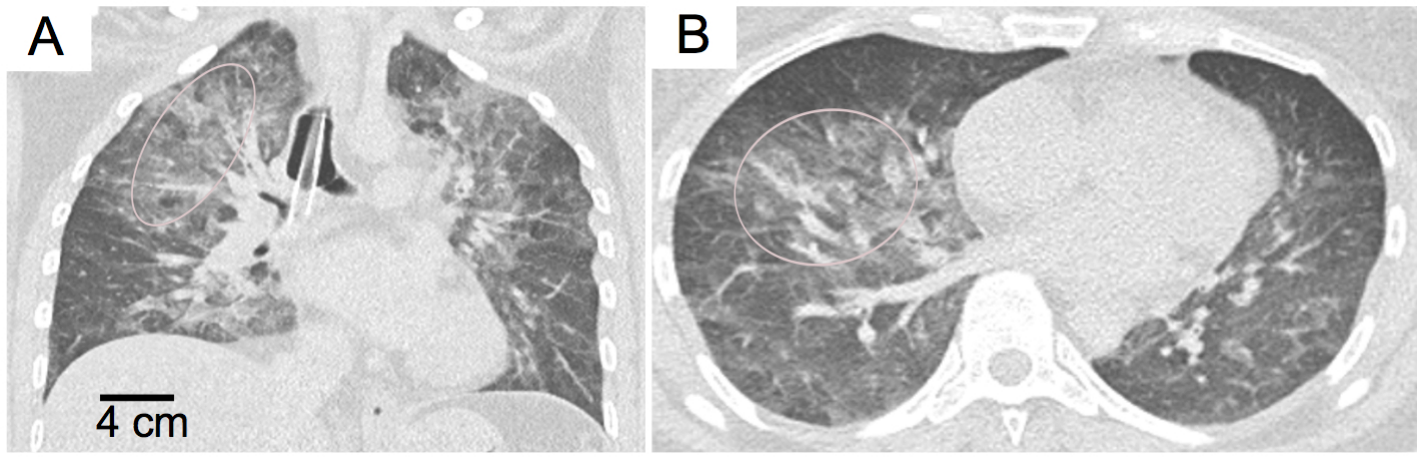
Computed tomography (CT) human chest images of a deceased body showing pulmonary edema due to cardiac failure. Coronal CT image (A) at a level dorsal to the tracheal bifurcation and axial CT image (B) of the lower lobes. The pulmonary edema indicated by the markers presents as diffuse ground-glass opacities with a predominant perihilar distribution. Both images: 3 mm slice thickness, window level -600 HU, window width 1700 HU.
